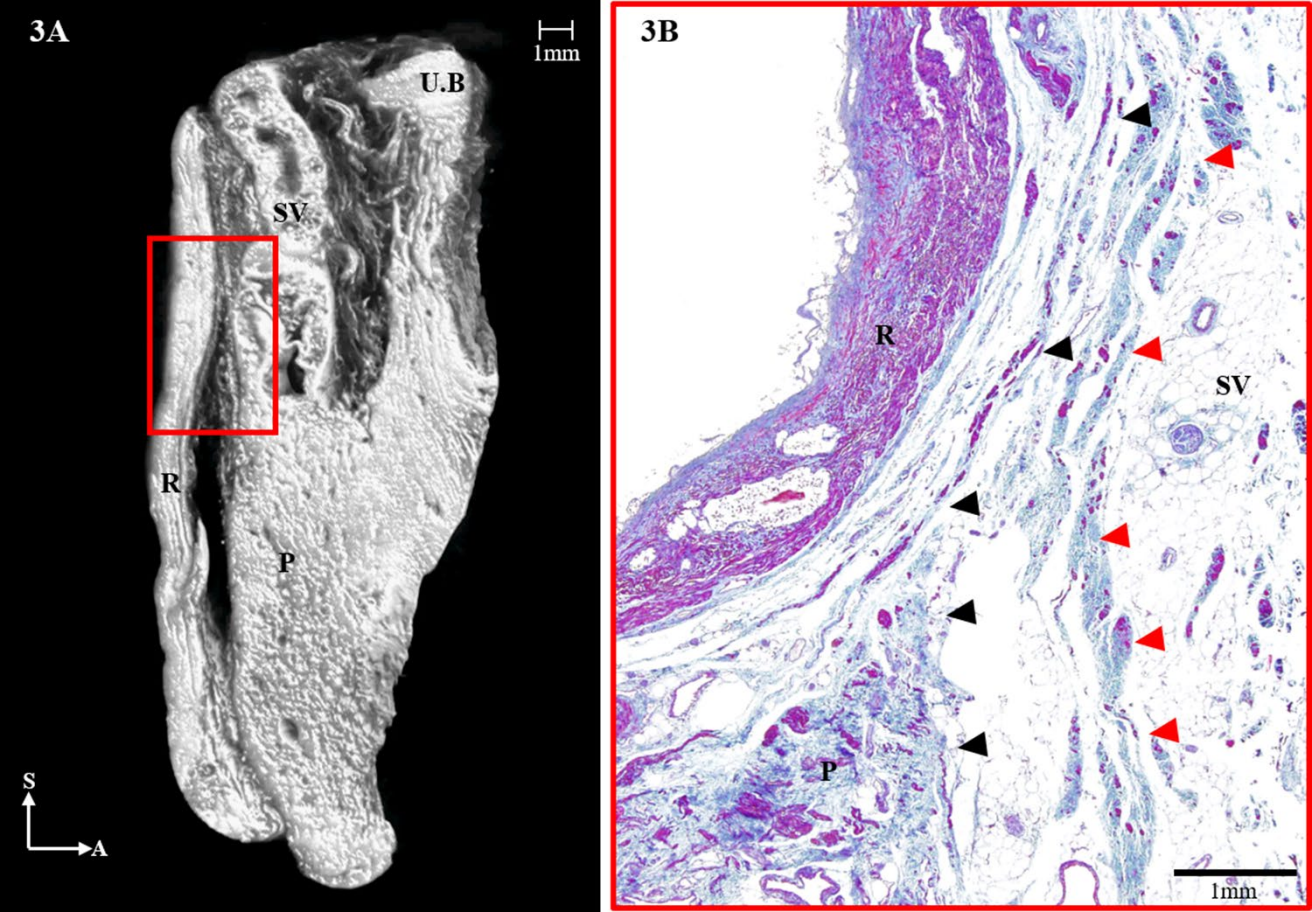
(A) Three-dimensional anatomy of the Denonvilliers’ fascia (DVF; red) from a sagittal section reconstructed from micro-computed tomography. (B) The distal, seminal part of the DVF is shown diverging to the anterior sheet and merging with the prostatic capsule (red arrowheads) and the posterior sheet (black arrowheads), covering the rectum. UB, urinary bladder; SV, seminal vesicle; P, prostate; R, rectum; S, sagittal; A, anterior.
